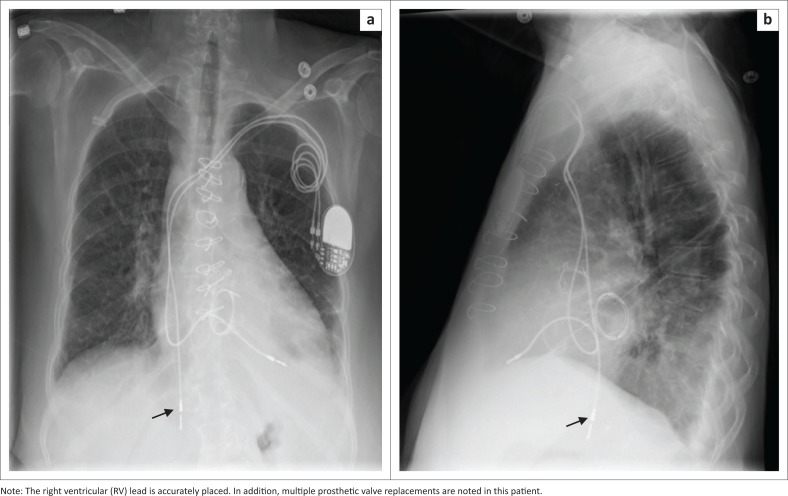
Frontal (a) and lateral (b) view chest radiographs (CXRs) showing a dual-lead pacemaker with the right atrial lead (arrow) abnormally positioned in the inferior vena cava.
